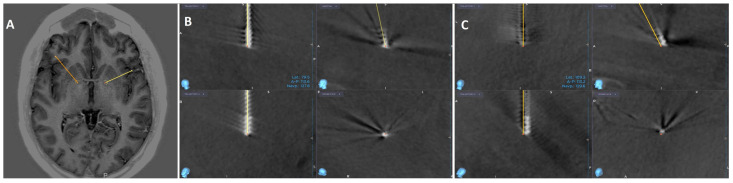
(A) Inverse recovery axial MR image of the planned targets for the first patient. (B,C) show the CT image after final lead placement for right (yellow) and left (orange) electrode, respectively, merged with the preoperative plan to confirm the final position. It is displayed in four different views: top left shows Trajectory 1, bottom left shows Trajectory 2, top right is the sagittal view, and bottom right is the probe’s eye view.
